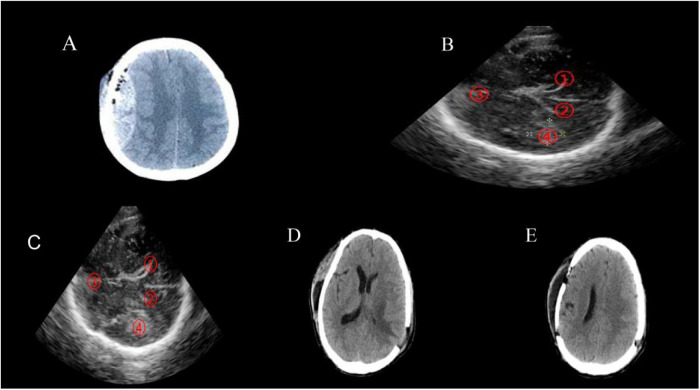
Brain CT image of case 1. (A,B) The patient was admitted to our hospital 1 h after the acute head injury. Brain CT showed a right temporoparietal epidural hematoma. (C,D) Ultrasonography of the brain was performed intra-operatively and the results showed a left temporal intracerebral hematoma [(1) the right posterior horn of the lateral ventricle. (2) the left posterior horn of the lateral ventricle. (3) the midline structure. (4) the hyper-echo area of the left temporal lobe might indicate the intracerebral hematoma of the left temporal lobe]. (E,F) After the skull was immediately closed and the family members of the patients were informed, the intracerebral hematoma was removed by small bone window craniotomy in the left temporal region. The pupil was contracted after surgery.
